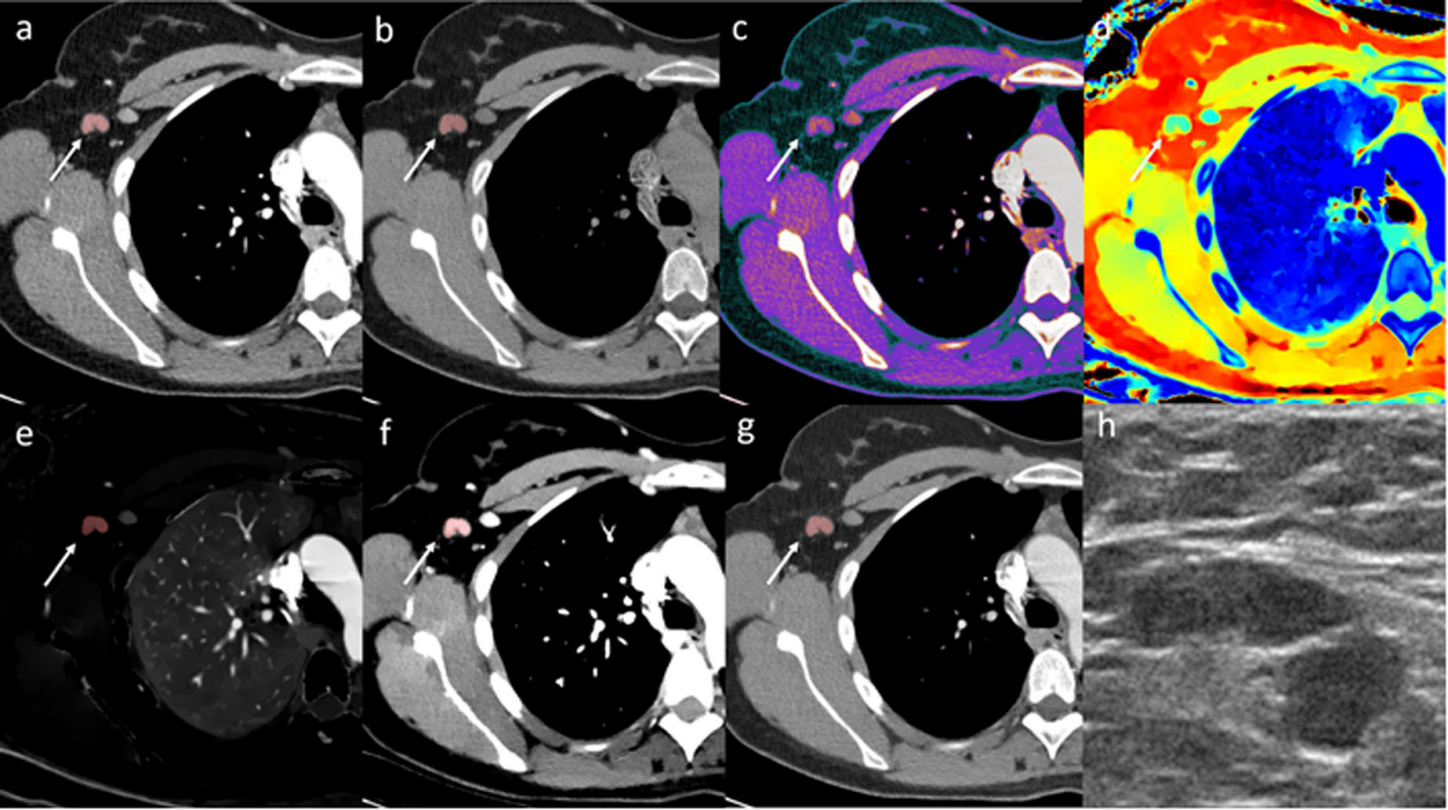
Arterial phase dual-layer detector spectral CT (DLSCT) examination of a woman with a suspicious lymph node in the right axilla. The lymph node shows high conventional CT HU values (a), Δ contrast enhancement (c) (conventional CT (HU) (a) − virtual non-contrast (HU) (b)), low Z effective (d), high iodine density (e), and spectral slope ((mono-energy 40 keV (f) minus mono-energy 100 keV (g))/60 keV). Based on the cutoff values, DLSCT diagnosed the lymph node as metastatic. Axillary ultrasonography (h) described the lymph node as suspicious; however, fine-needle aspiration was negative. Sentinel lymph node biopsy contained malignant tumour cells from invasive ductal carcinoma
